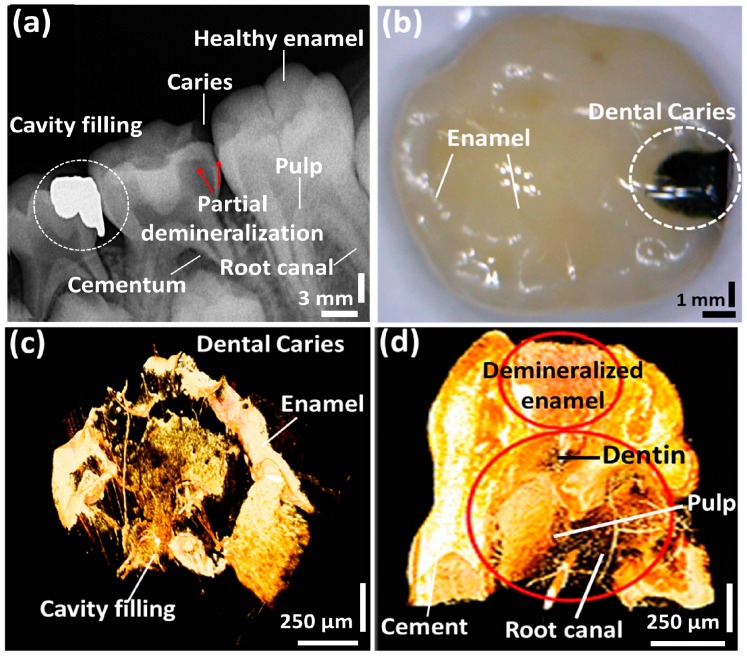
Structural analysis of the carious tooth sample using various inspection methods. (a) Radiographic image of the carious tooth sample before the surgery; (b) Photograph of the carious tooth sample after the surgery; (c,d) top and side views of 3D OCT images of the carious tooth sample.
